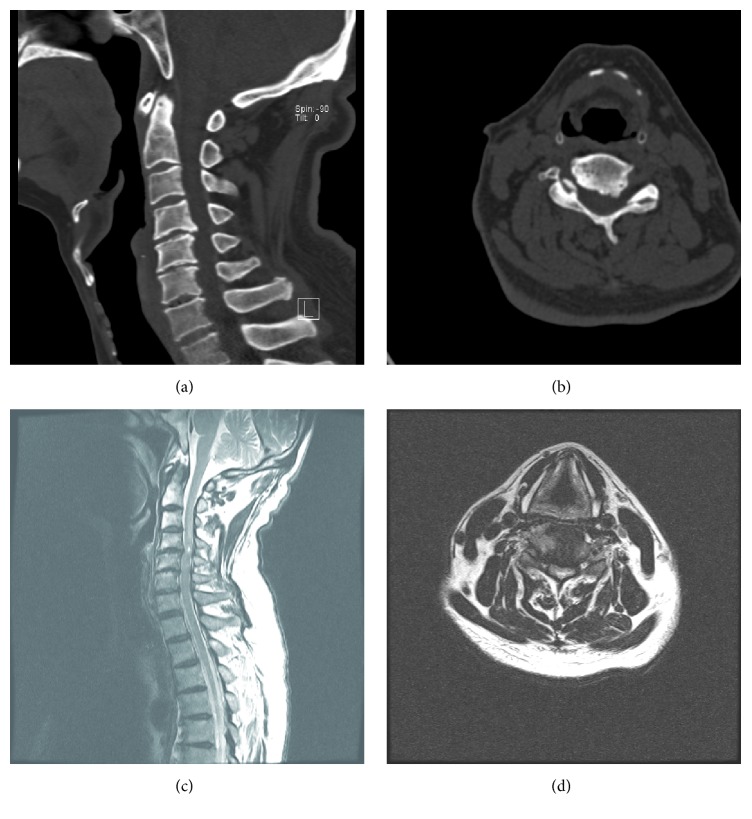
Imaging studies obtained in a 69-year-old man injured in a high falling accident. ((a) and (b)) Preoperative CT showing obvious osteophytes of C4-5. (c) and (d) MR image demonstrating C4-5 cord compression and an intramedullary high signal intensity.
